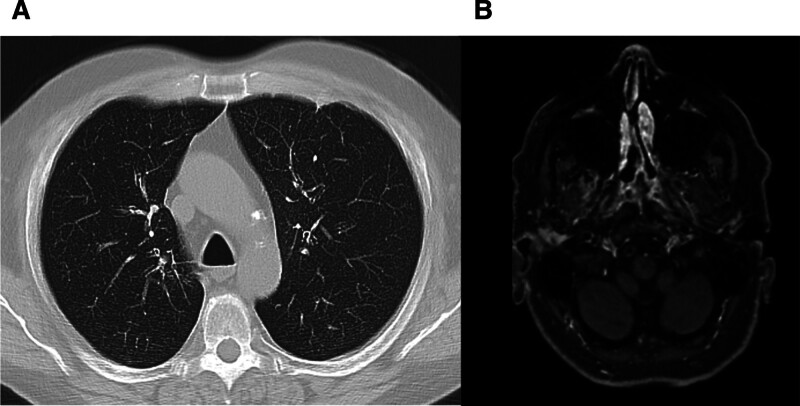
Posttreatment imaging assessment: (A) thoracic CT-scan showing the disappearance of the pulmonary nodule; (B) cranial MRI showing clear regression of the ENT lesion.
